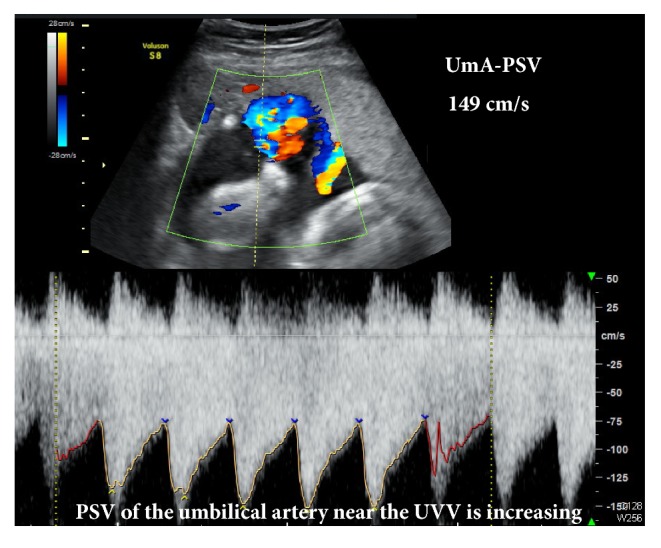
Ultrasonographic echocardiogram at 32 weeks of gestation shows edematous enlargement of the umbilical vein varix and an increase of the peak systolic velocity of the umbilical artery (UmA-PSV) up to 149 cm/s.
